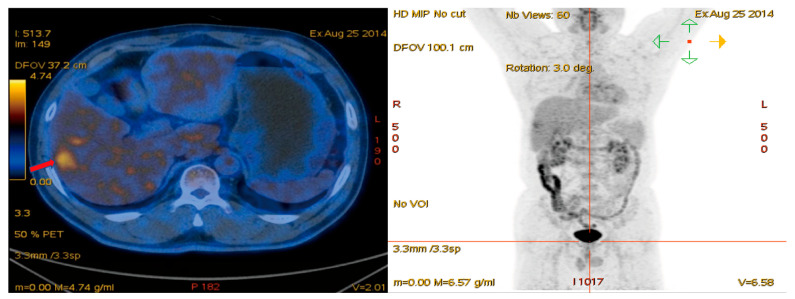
Representative imaging and the calculation of parameters in a patient with HCC. In this example, the TSUV_max_ is 4.13, the LSUV_mean_ is 2.3, and the TLR is 1.80. HCC, hepatocellular carcinoma; LSUV_mean_, average standardized uptake value in normal liver tissue; TLR, tumor-to-liver ratio; TSUV_max_, maximum standardized uptake value in tumor tissue.
